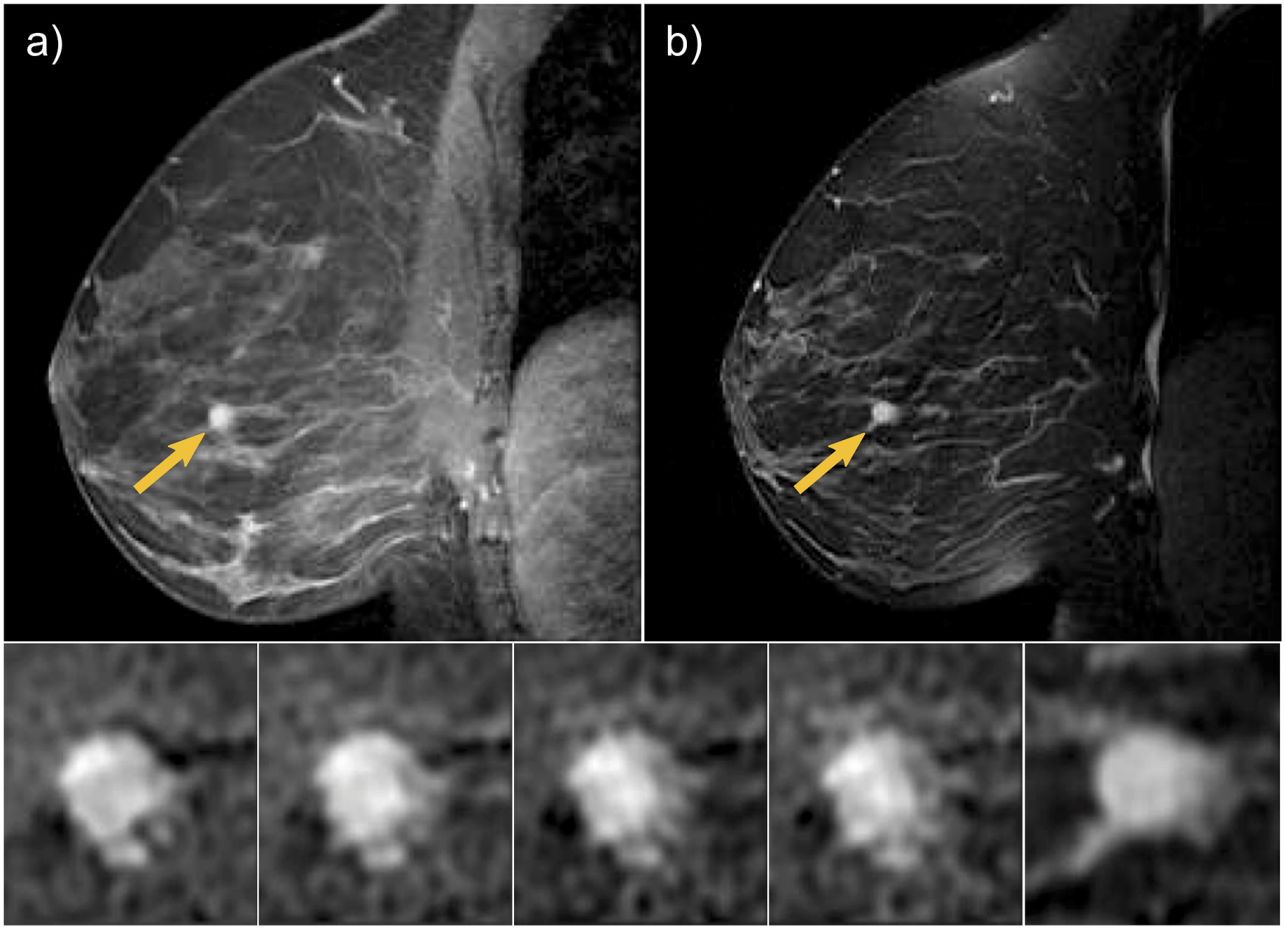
A benign fibroepithelial lesion with fibrocystic changes and hyperplasia in a 35-year old high risk woman. The lesion appeared as a circumscribed round mass, with smooth margins, rapid early enhancement and mild washout in delayed enhancement (BI-RADS score 4). a) T1w 4th post-contrast DCE-MRI. b) T2w MIR. c) zoomed progressive enhancement at each of the four post-contrast scans. d) zoomed lesion T2w appearance.
